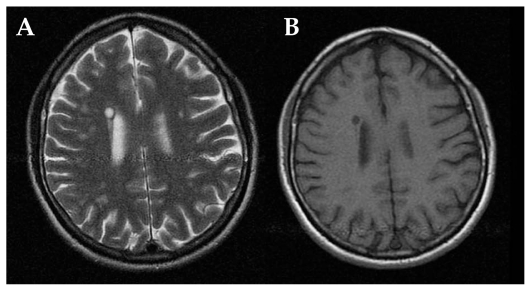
MRI in multiple sclerosis: a hyperintense parietal white matter lesion on T2 (A), appears hypointense on T1 images (B).
